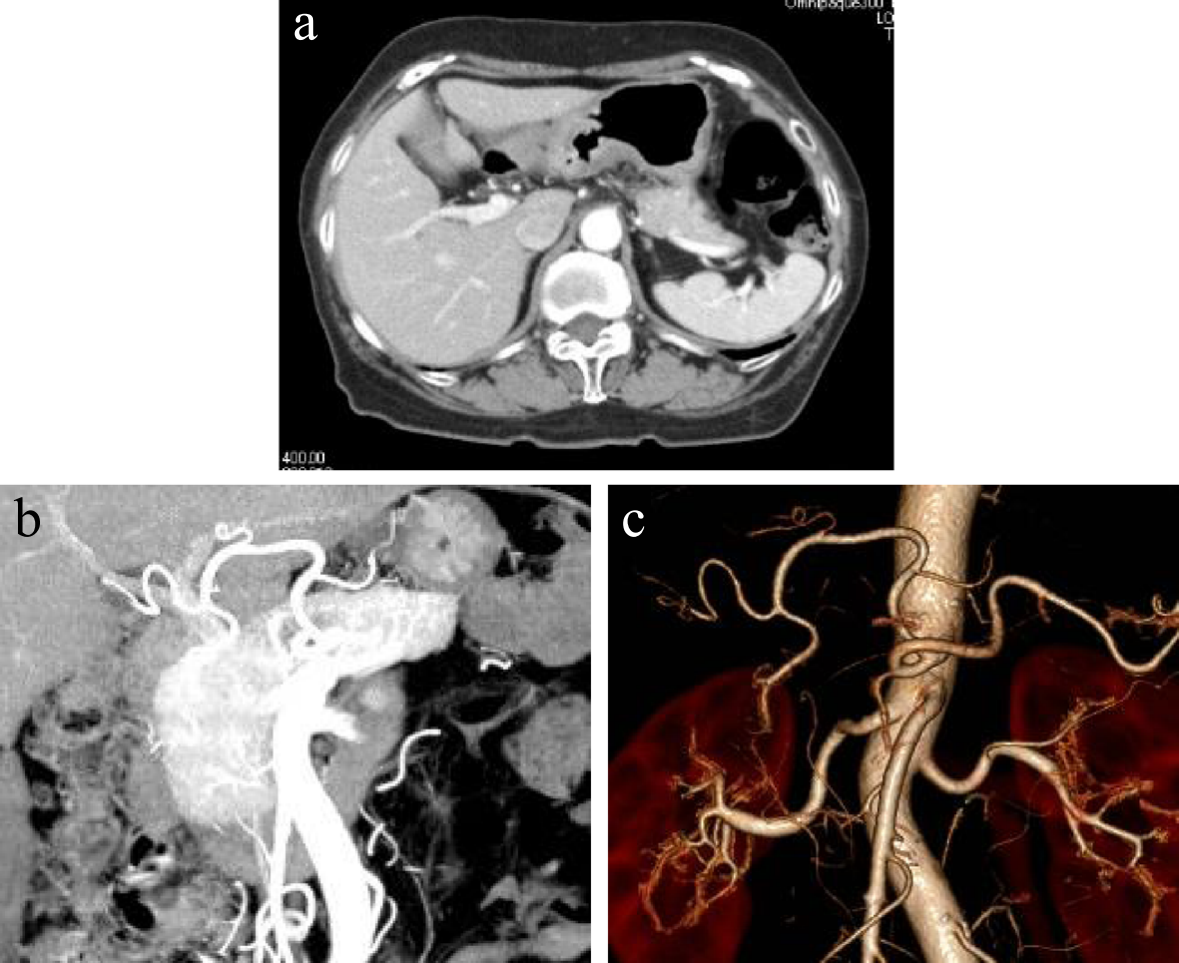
Findings of the enhanced computed tomography scan. a Wall thickening of the stomach that was a suspected invasion of the subserosa. b, c:The dynamic computed tomography and computed tomography angiogram showed the ectopia of the common hepatic artery branched from the left gastric artery
